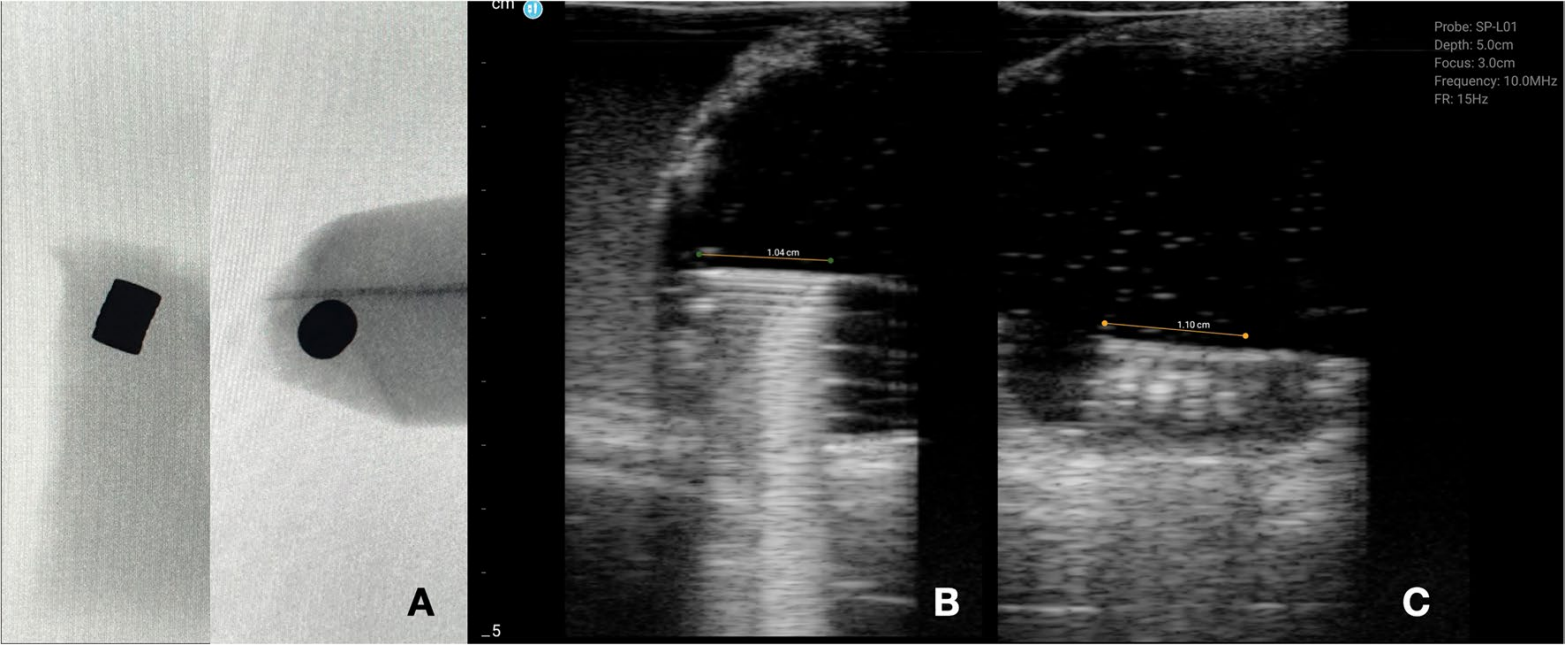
Stacked disc magnets. A Radiographic appearance of stacked disc magnets in longitudinal and en face configuration. B Transverse and (C) longitudinal views on sonography of the stack of magnets in horizontal orientation. Note the hypoechoic lines marking the interface of each magnet
